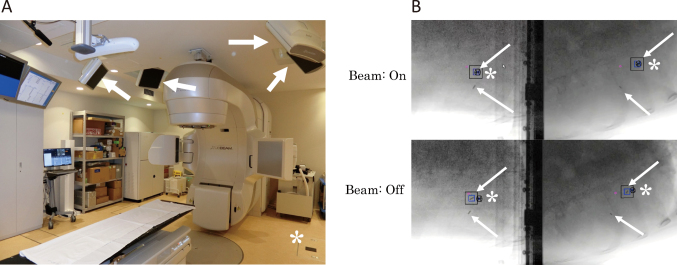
(A) The tracking system on a medical linear accelerator (Linac). The white arrows indicate X-ray detecting boards and the asterisk indicates one of four X-ray sources placed under the floor. (B) We usually use two sets of X-ray systems to tract a gold fiducial marker placed near the target volume. While the marker is out of position, the Linac beam is off. If the marker moves into the predefined position on images from both directions, the radiation beam is on.
